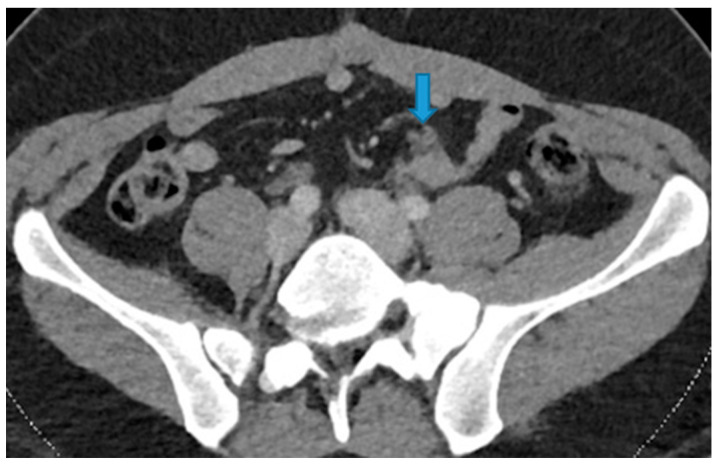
Axial CE-CT. PC from colon adenocarcinoma: nodular deposit within the mesentery (arrow).
